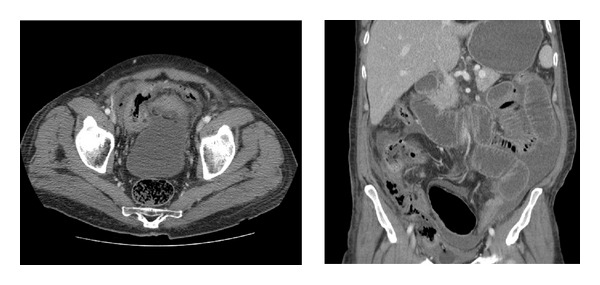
Control computed tomography scan of the abdomen showed necrosis of the bladder wall, predominantly the bladder dome and right bladder wall, with associated peritonitis signs.
